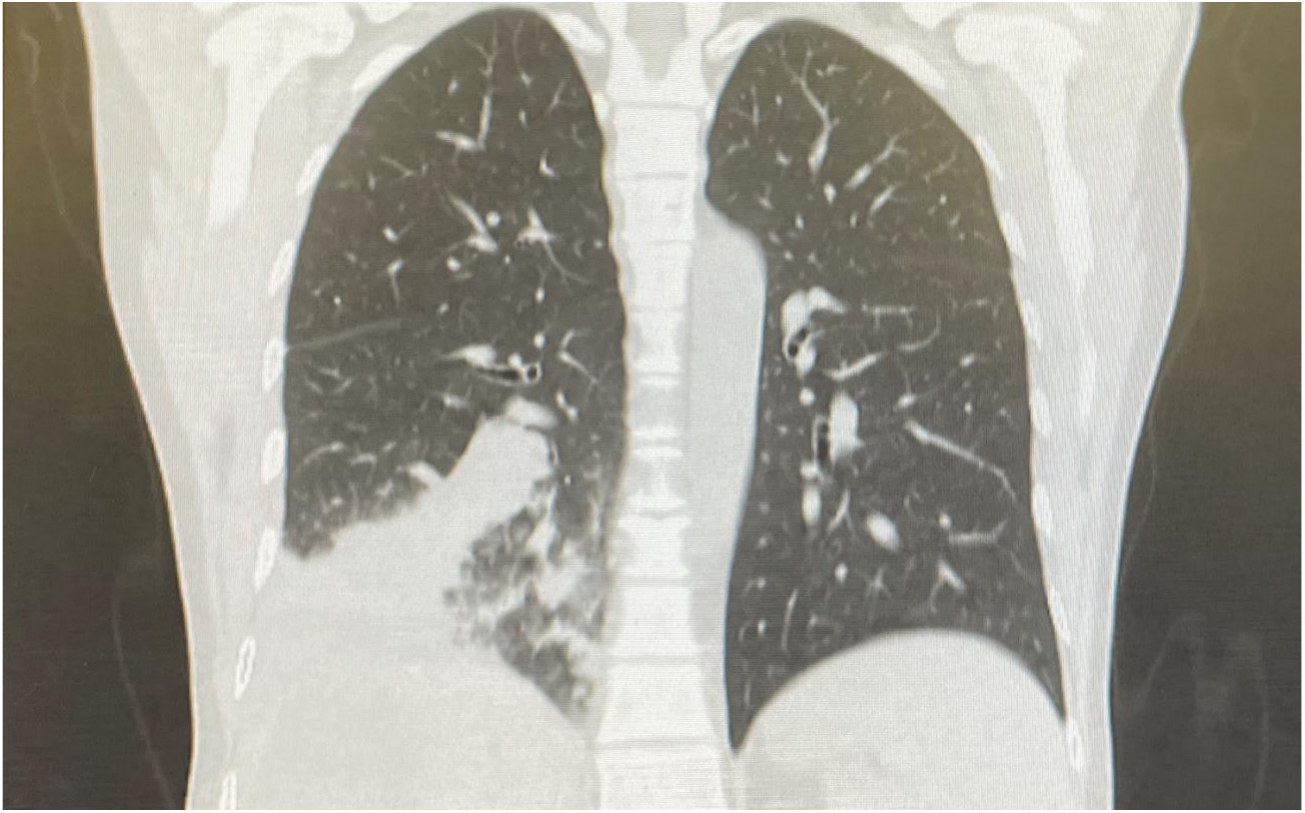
Coronal view of a preoperative chest computed tomography scan of our 45‐year‐old case with bronchobiliary fistula.
